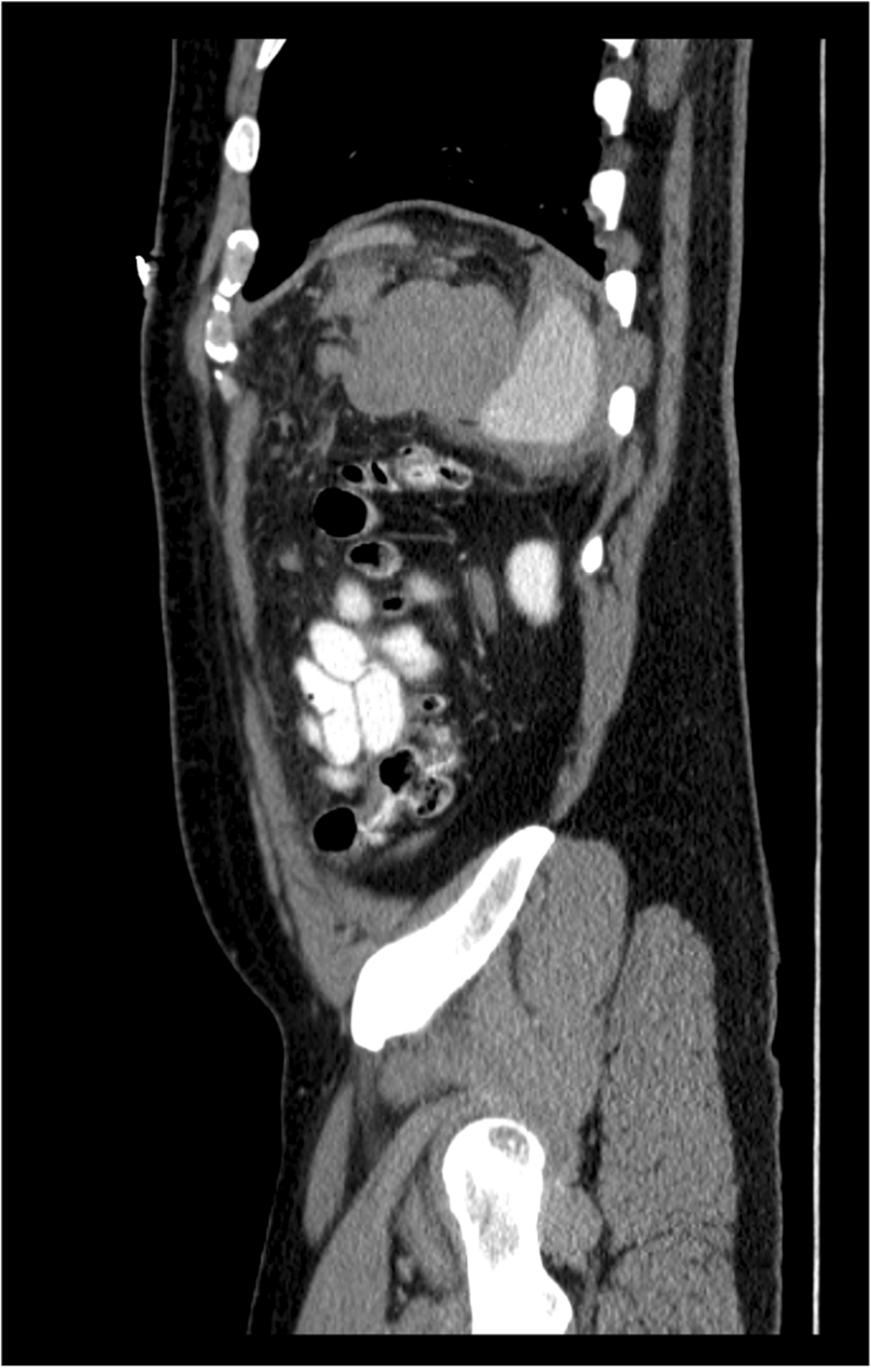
Sagittal, contrast-enhanced CT image demonstrates perisplenic hematoma.
